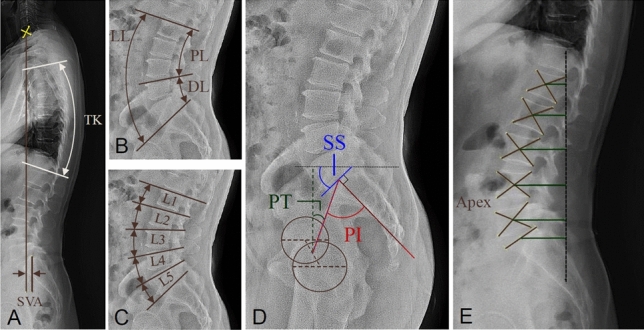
Representation of radiographic parameters. (A) TK denotes global thoracic kyphosis, SVA denotes sagittal vertical axis. (B) LL denotes lumbar lordosis, PL denotes proximal lumbar lordosis, and DL represents distal lumbar lordosis. (C) Segmental lordosis. (D) PI denotes pelvic incidence, PT represents pelvic tilt, and SS denotes sacral slope. (E) Lumbar apex. The maximum distance between the center of the vertebrae and the gravity line.
